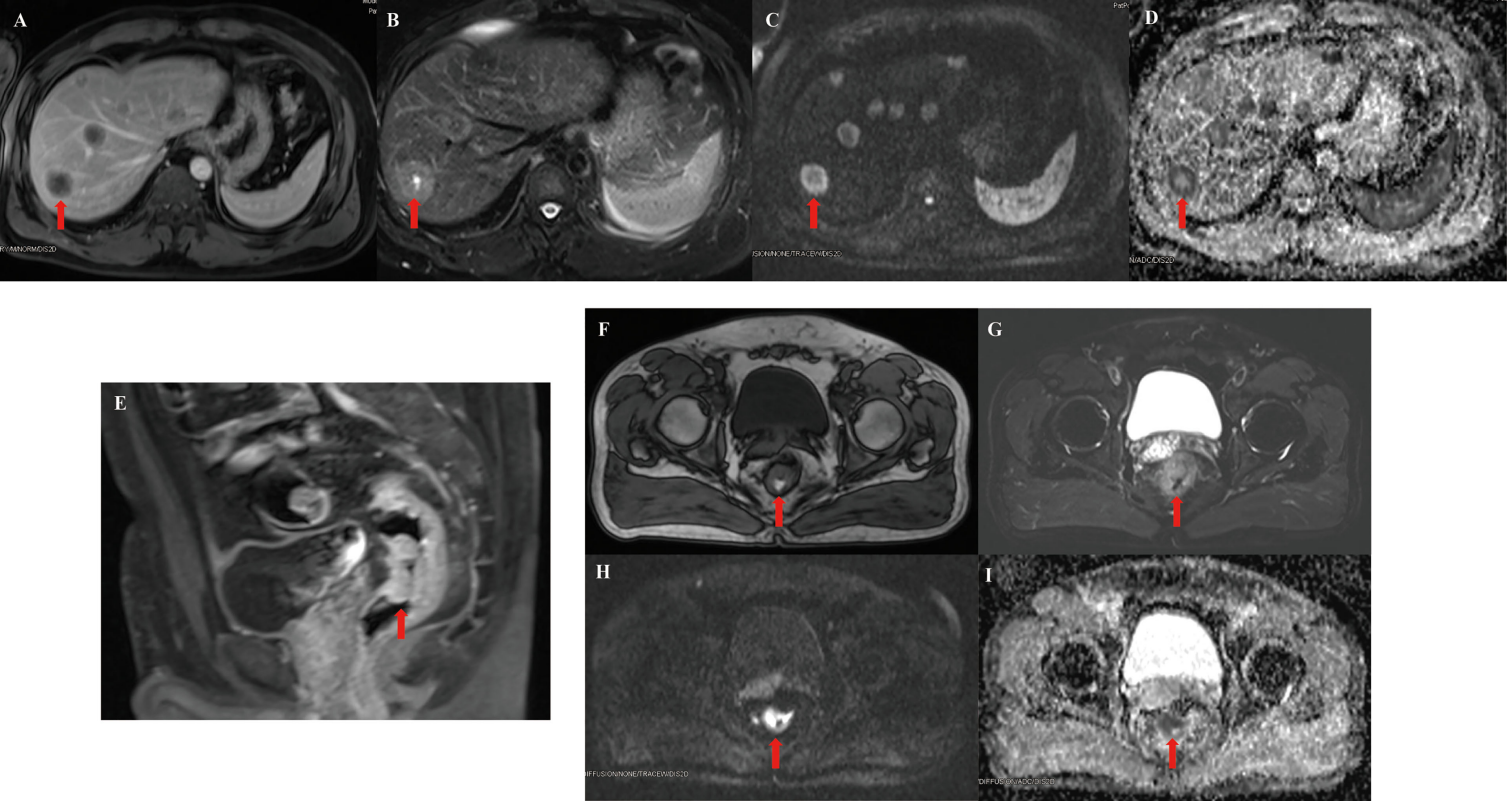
Representative magnetic resonance imaging (MRI) images of lesions. (A–D) Representative images of T1-weighted imaging (T1WI), T2-weighted imaging (T2WI), diffusion-weighted imaging (DWI), and apparent diffusion coefficient (ADC) in the liver. (E–I) Representative images of rectum lesions in the sagittal position and coronal position (T1WI, T2WI, DWI and ADC), respectively. Red arrows indicate typical liver or rectum lesions.
